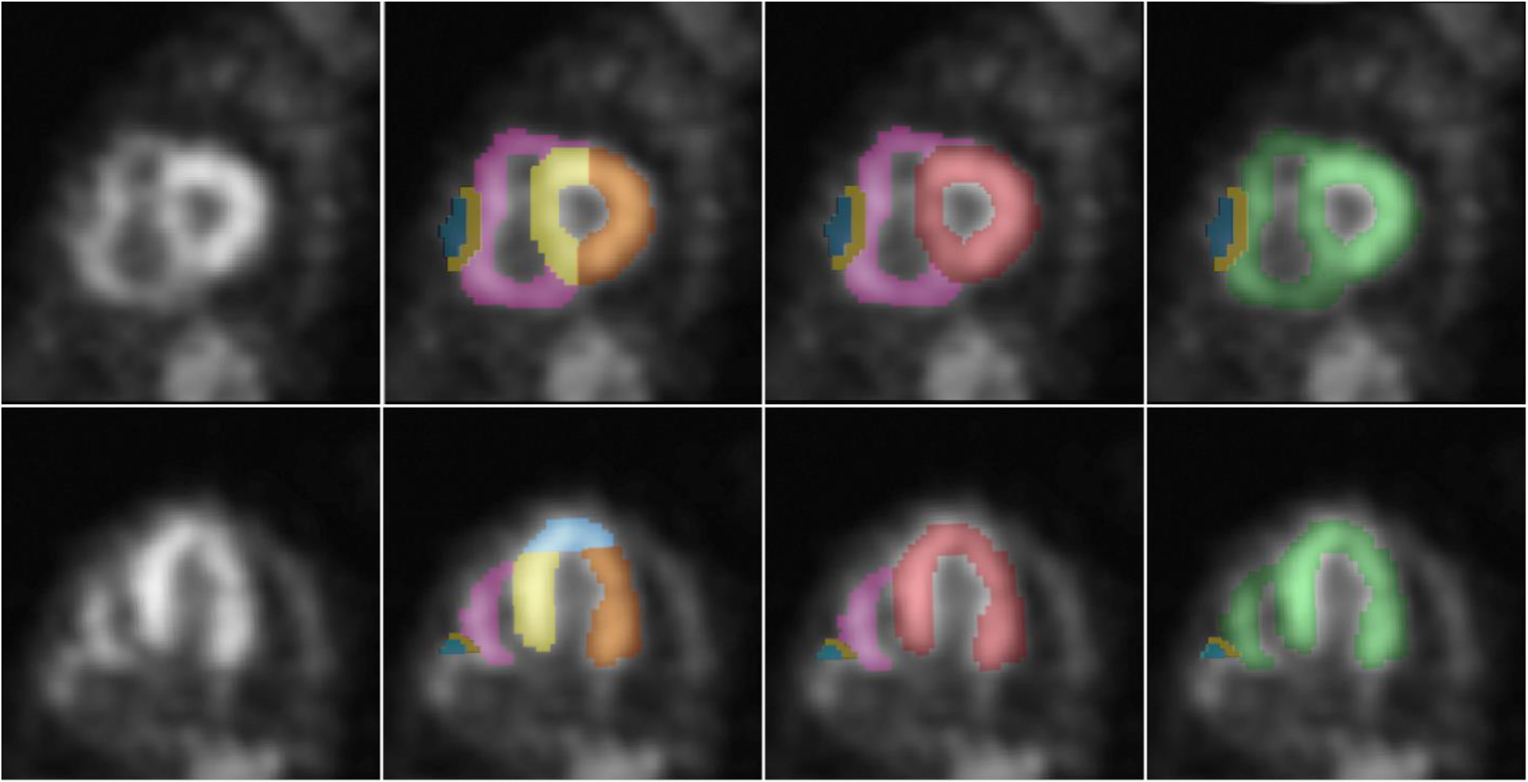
DPD-SPECT slices in apical short axis view (top panels) and apical long axis view (bottom panels) demonstrating volumes of interest (VOIs) mapping and sternum isolation from the right ventricular wall for minimizing the sternal activity spill-over. Different VOIs are presented in colours as defined in Fig. 1. Purple—right ventricular free wall (RV-free-wall); yellow—interventricular septum (IVS); blue—apex of the heart (Apex); orange—left ventricular lateral wall (LV lateral wall); red—left ventricle (LV); green—heart ventricles (whole heart); mustard yellow—boarder around sternum; turquoise—sternum spill over
